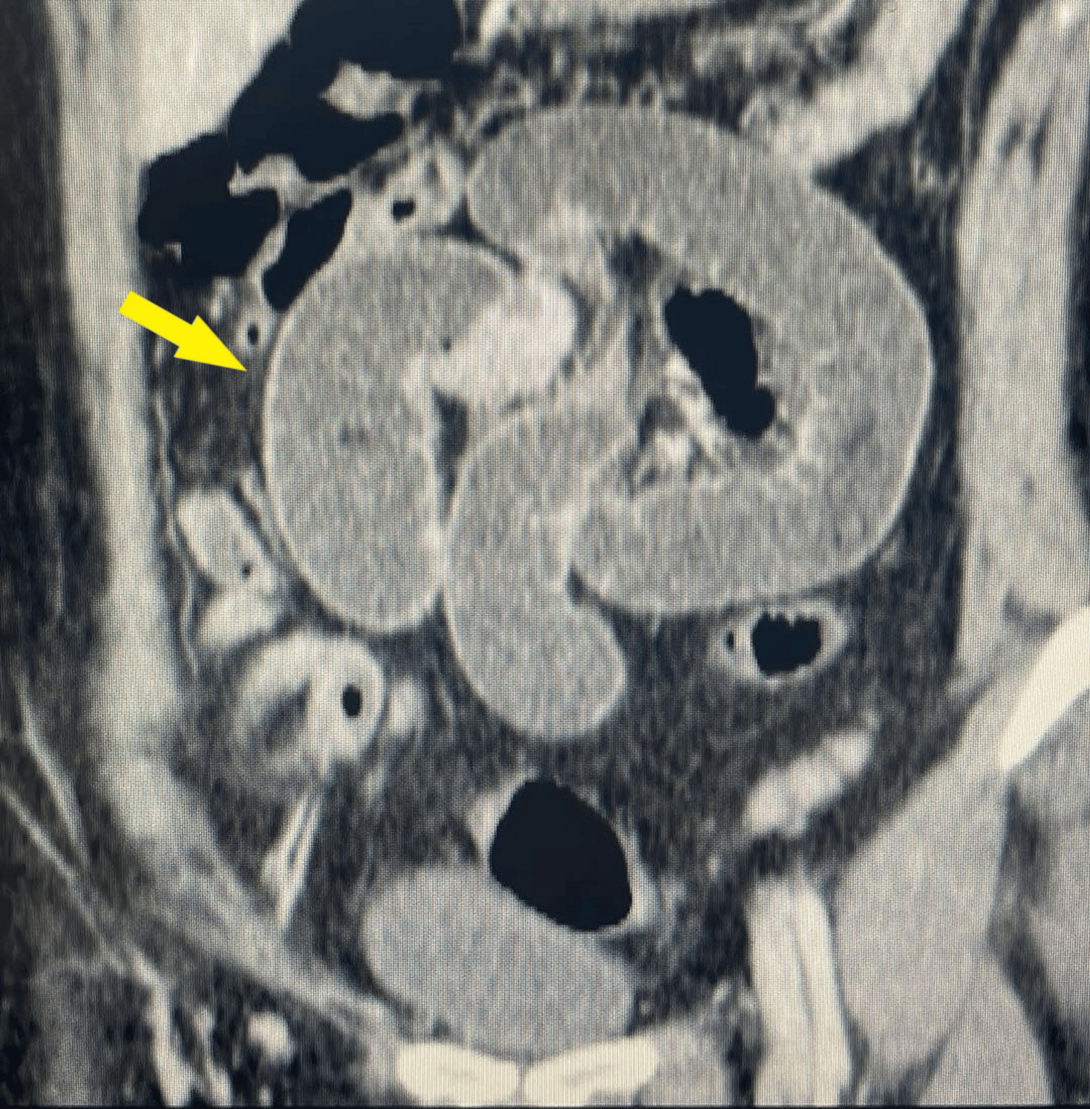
CT scan showing dilated loops of the the small intestine (yellow arrow).
